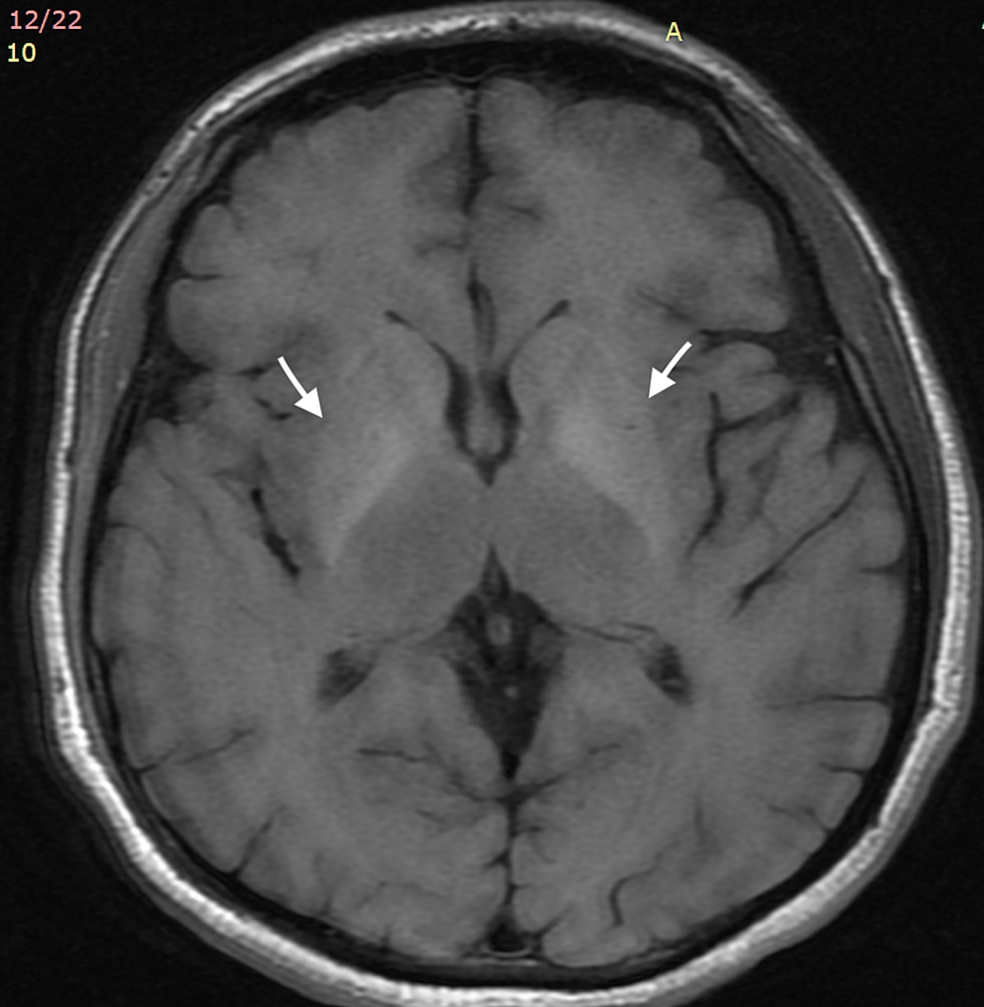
Axial T1-weighted MRI of the brain. MRI of the brain showing symmetrical hyperintensity on T1-weighted images in the globus pallidus (arrows).
